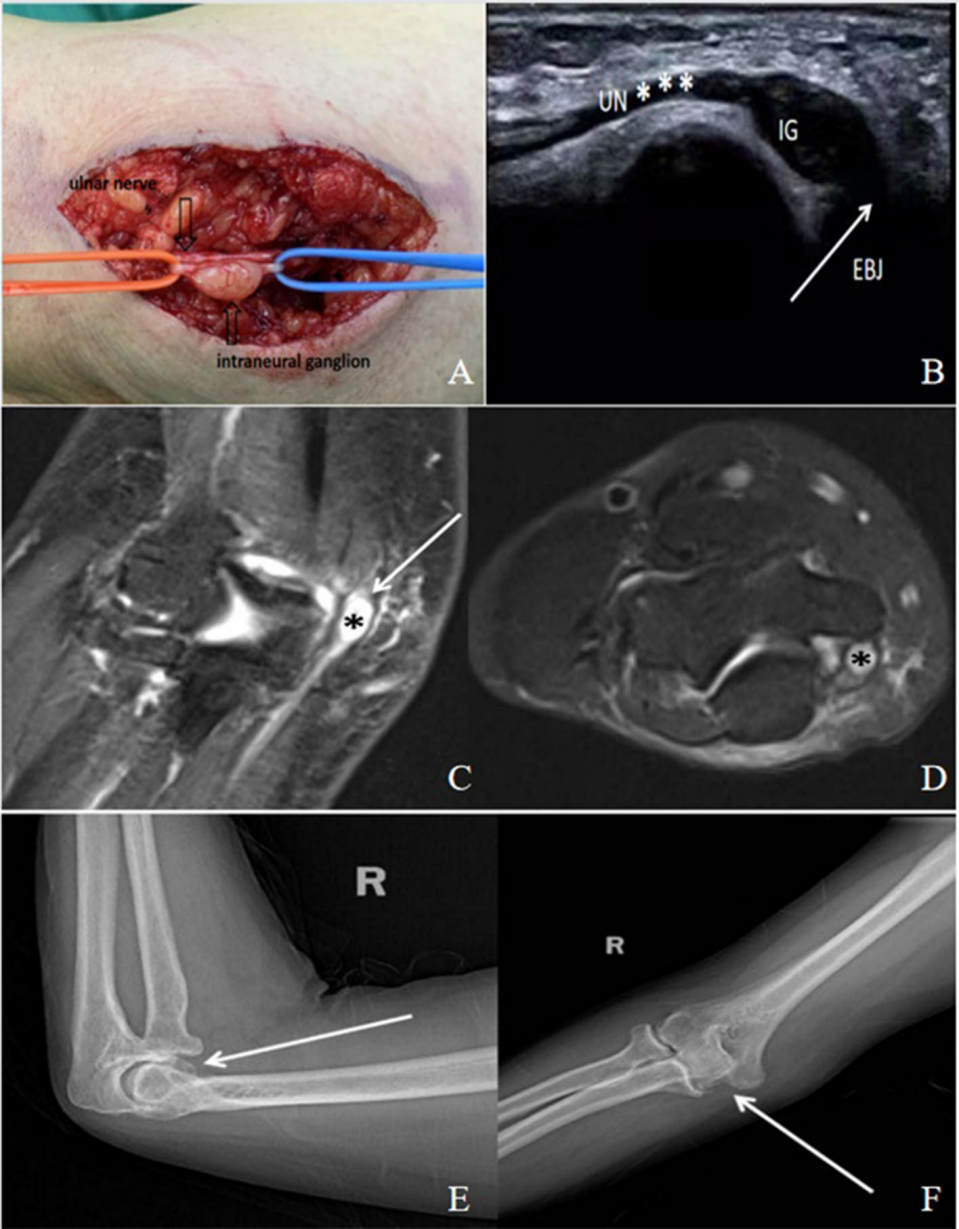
A 45-year-old male patient with cubital tunnel syndrome caused by intraneural ganglion (IG). (A) An intraneural ganglion (IG) was found within the ulnar nerve (UN*) in the cubital tunnel. (B) An ultrasound image shows an intraneural cyst extending along the ulnar nerve. EBJ: elbow joint. (C,D) There was a close link between the source of the intraneural cyst and the elbow joint, which is shown in the MRI (arrow). The intraneural ganglion (*) is shown as (C), a high signal in T2STIR in sagittal view, and (D) a high signal in T2STIR in transverse view near the elbow joint. (E,F) Anteroposterior and lateral X-ray films of the elbow joint, showing the existence of elbow arthritis (arrow).
